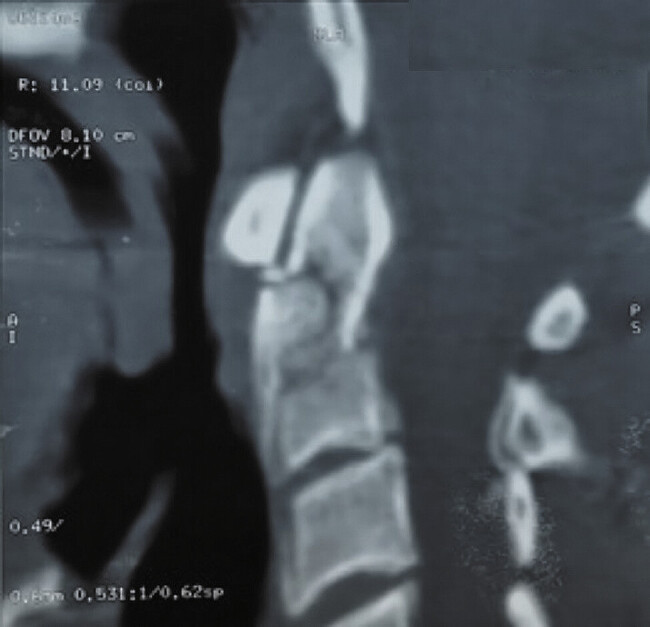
Cervical computed tomography with simple reconstruction. Comminuted fracture of the odontoid process of the second cervical vertebra.
